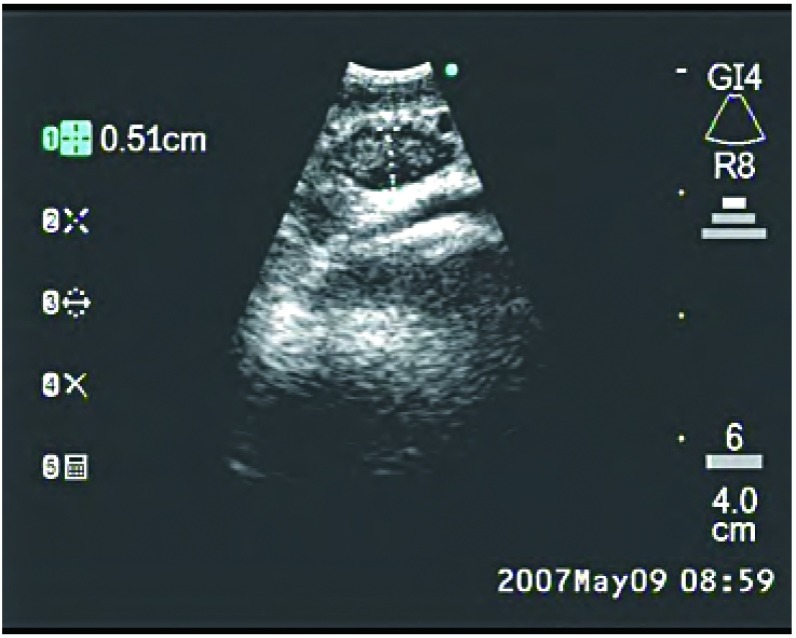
支气管内超声所见气管隆突下淋巴结 Endobronchial ultrasound of subcarinal node
